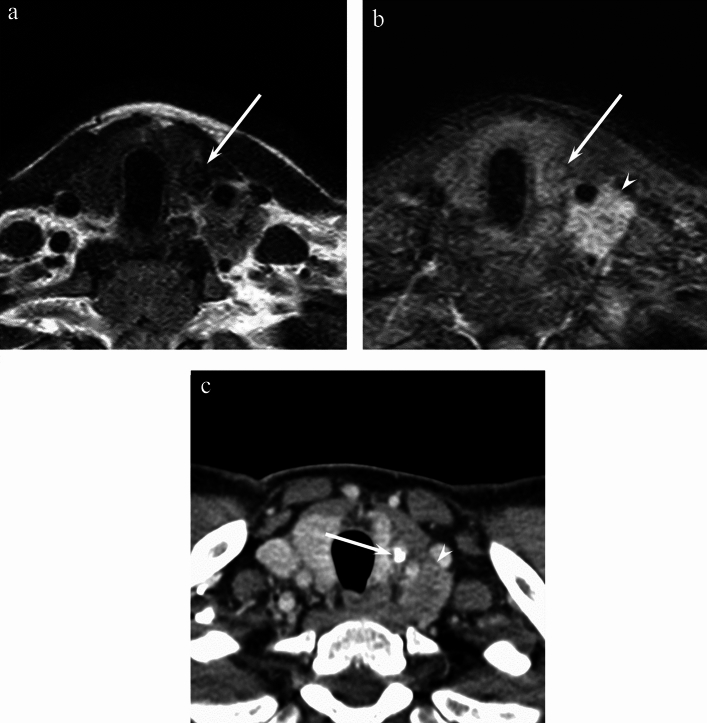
A 38-year-old man with papillary thyroid carcinoma of the left lobe. a T2-weighted image (TR/TE, 3456/90 ms) shows a hypointense mass (arrow). b Fat-suppressed contrast-enhanced T1-weighted image (TR/TE, 675/17 ms) shows mild enhancement (arrow). The tumor shows a well-defined margin without adjacent organ invasion but left lower jugular lymph node metastasis (arrowhead) is observed. c Contrast-enhanced CT image shows intratumoral nodular calcification (arrow) and left lower jugular lymph node metastasis (arrowhead)
